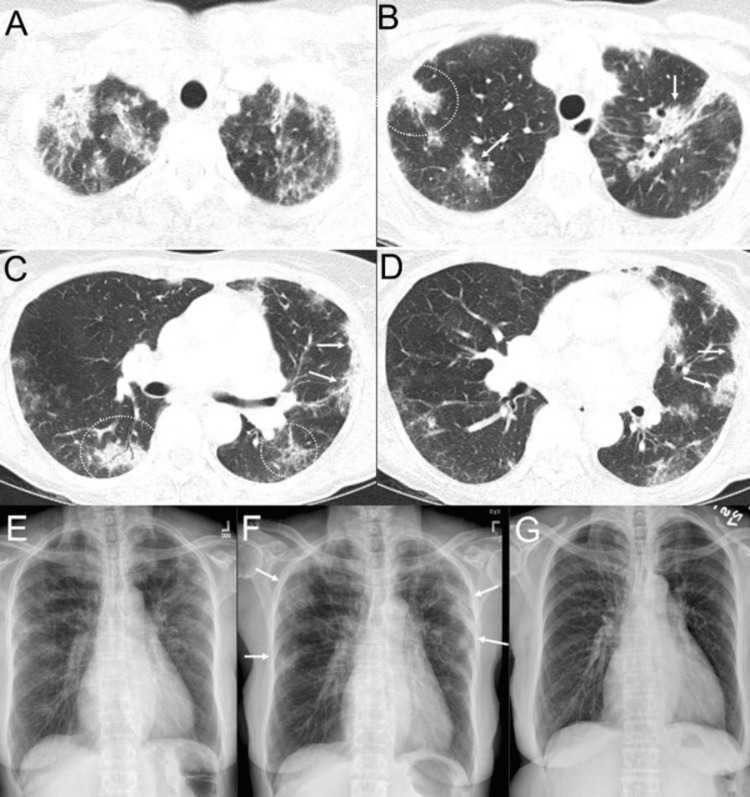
Chronic eosinophilic pneumonia in a patient presenting with chronic cough, wheezing, worsening radiographic lung opacities, and peripheral eosinophilia. Axial CT images (A-D) show bilateral, multifocal upper lobe predominant and peripheral to a lesser extent peribronchial mixed ground-glass opacities and consolidations associated with mild reticular opacities (some areas annotated with arrows and dotted circles). A frontal chest radiograph obtained one month prior to the CT (E) shows upper lobe and peripheral predominant hazy lung opacities with small consolidations. A frontal chest radiograph obtained a few days prior to the CT (F) shows persistent and increased lung opacities (arrows). The patient underwent bronchoalveolar lavage, which revealed an eosinophil count of 25%. A frontal chest radiograph obtained a few months after the CT (G), following steroid treatment, shows resolution of lung opacities.
